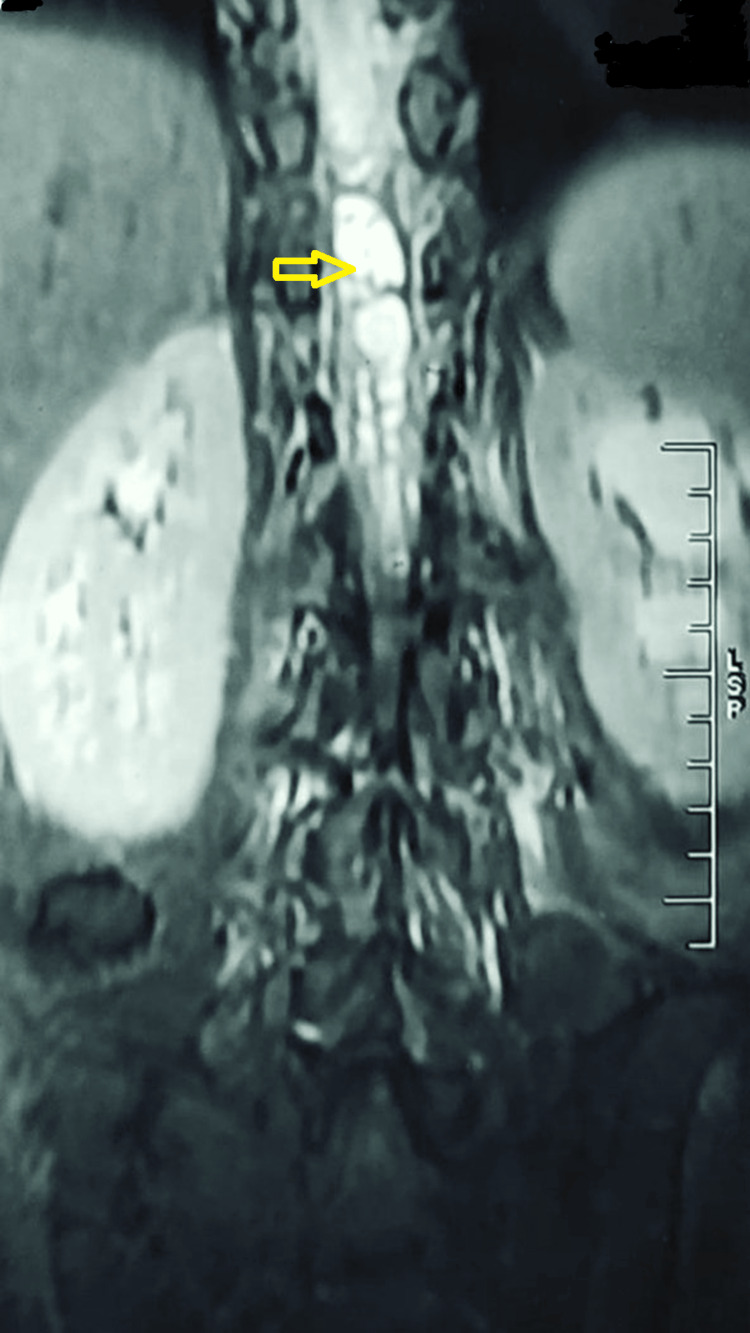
An MRI of the thoracolumbar spine (mid-coronal image) Loculated fluid collection with contrast enhancement in the spinal canal extending from T9 to T12 vertebral levels (yellow arrow) involving the distal cord, conus medullaris, and cauda equina nerve roots
